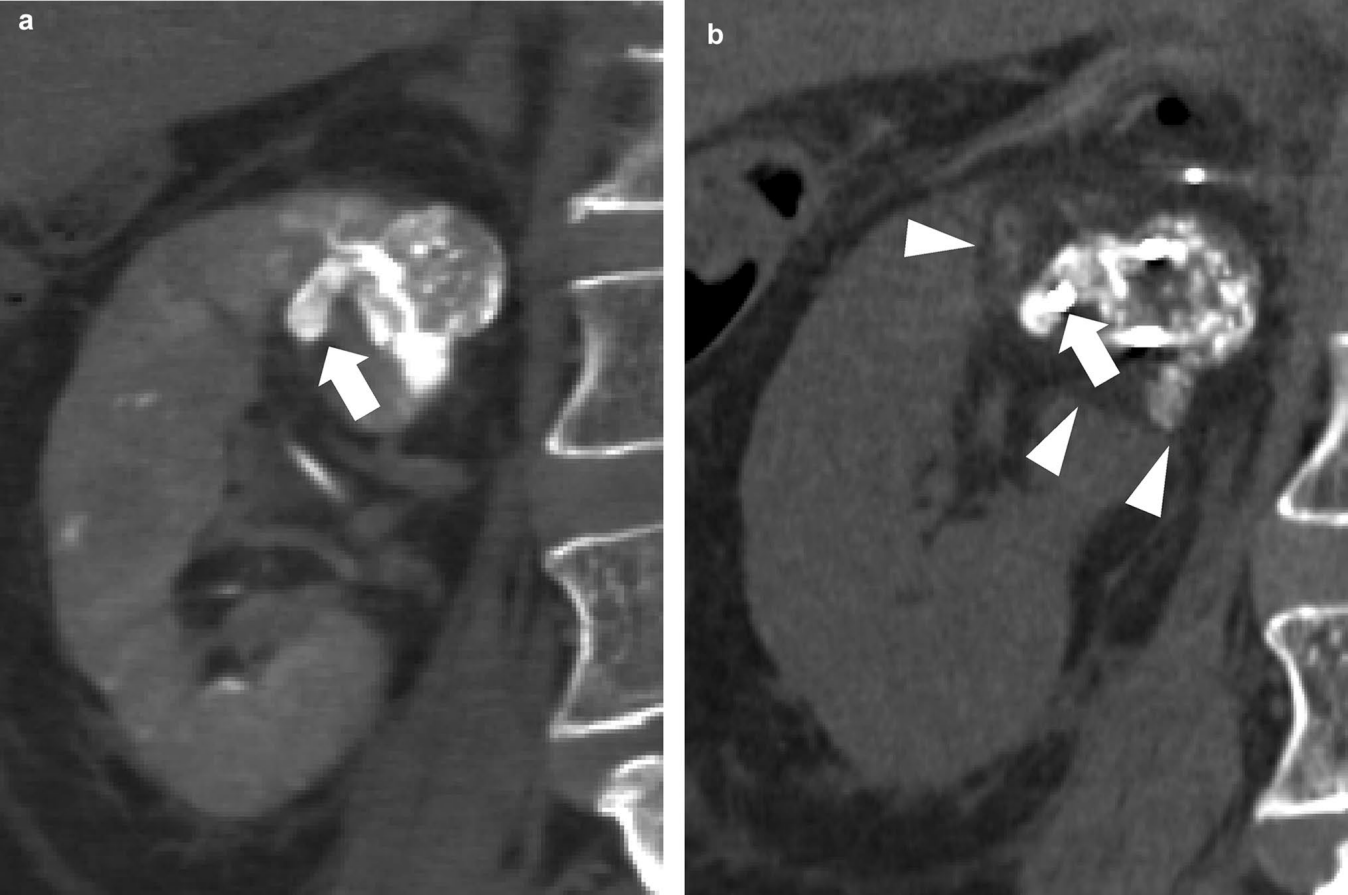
CT images of 2.9 cm T3a RCC in the right kidney in a 77 year-old man. a Coronal CT image immediately after TAE shows lipiodol accumulation in RCC, including the extension into the segmental vein (arrow). b Coronal CT image during ablation shows a cryoprobe directly inserted into the extension part into the segmental vein (arrow) and ice ball (arrowhead). This RCC achieves complete response. *CT* computed tomography; *RCC* renal cell carcinoma; *TAE* transcatheter arterial embolization
